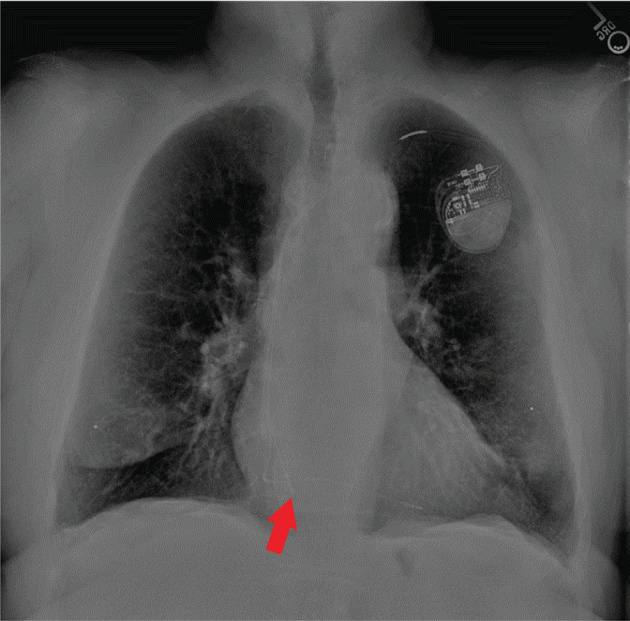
Chest radiograph showing a dual-chamber pacemaker, with the tip of a chronically dislodged atrial lead (red arrow) on the floor of the right atrium.
